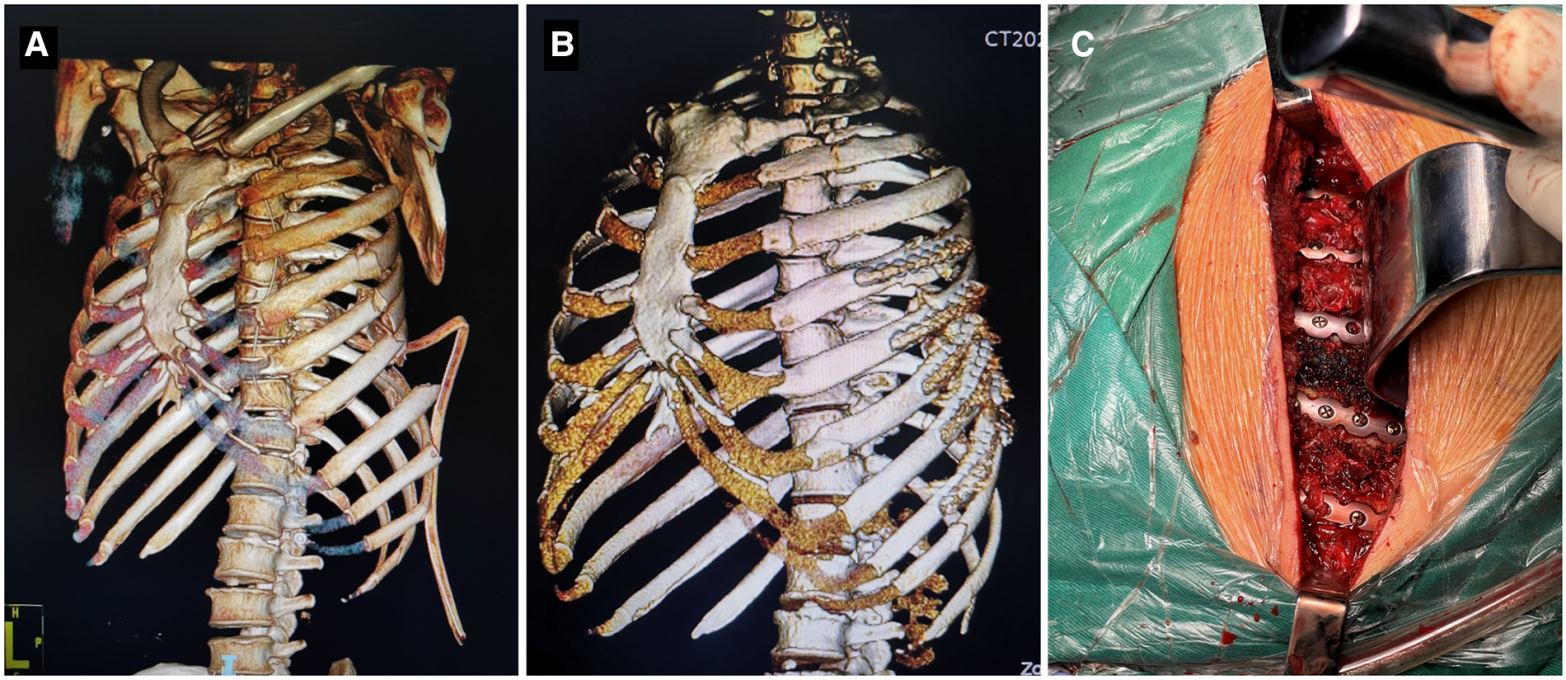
Internal fixation of a multiple rib fracture. A 57-year-old male with multiple traumas: traumatic hemothorax; flail chest with fractures of the left ribs 1–10 and right rib 5; bilateral pulmonary infection; bilateral pulmonary contusions. (A) CT 3D reconstruction prior to surgery suggested multiple fractures ribs. (B) Postoperative CT 3D reconstruction suggested that the multiple fractures ribs were repositioned and the internal fixation was well positioned. (C) Intraoperative photo showing after placement of internal fixation.
